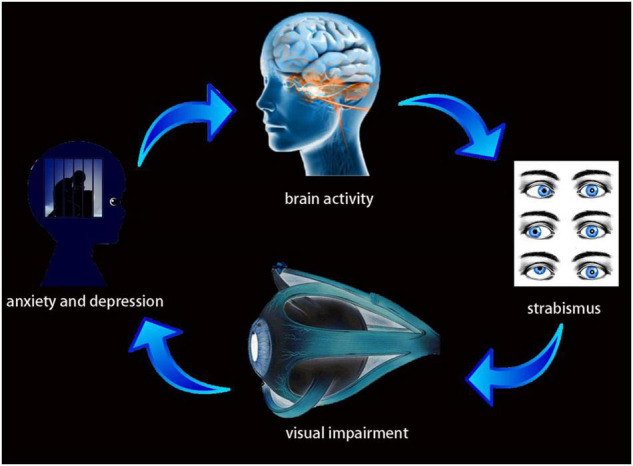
Relationship between MRI images and clinical manifestations in CS. Strabismus results from extraocular muscle dysfunction, leads to visual impairment, further result in anxiety and depression and alterations in brain activity.
